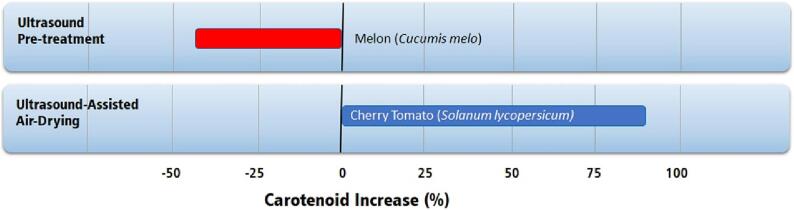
Highest increase in total carotenoids in fruits by applying ultrasound technologies compared to conventional air-drying [94], [124].
